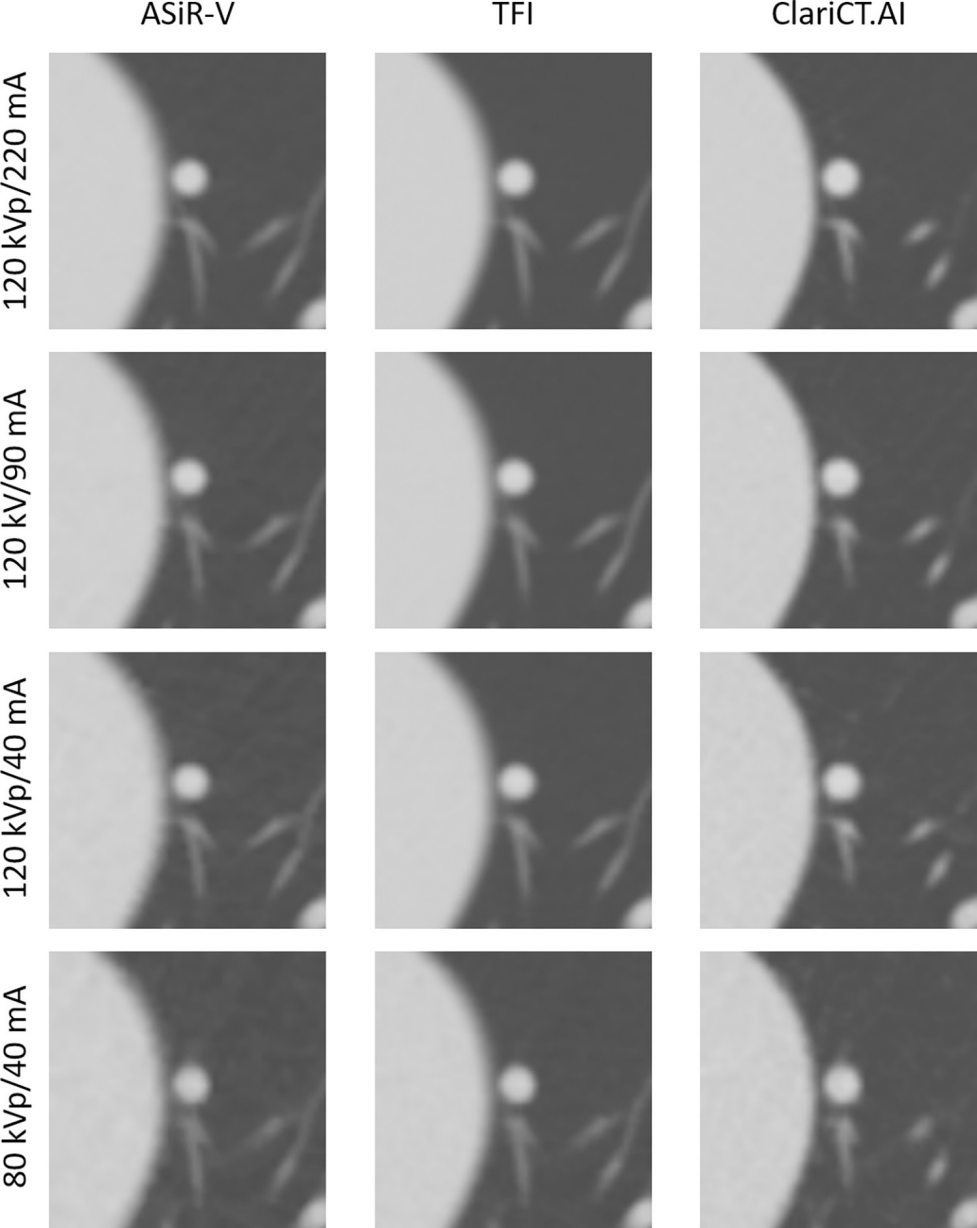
CT images of SNs at different radiation dose settings. Images of SNs (5 mm) at four radiation dose settings reconstructed with ASiR-V, TFI, and ClariCT.AI.
